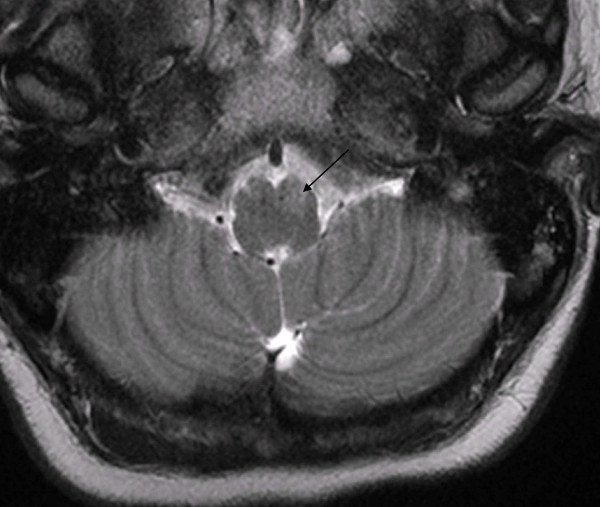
Transverse T2-weighted magnetic resonance imaging (MRI) scan of the posterior cerebral fossa showing symmetric signal intensity within the medulla oblongata before treatment.
